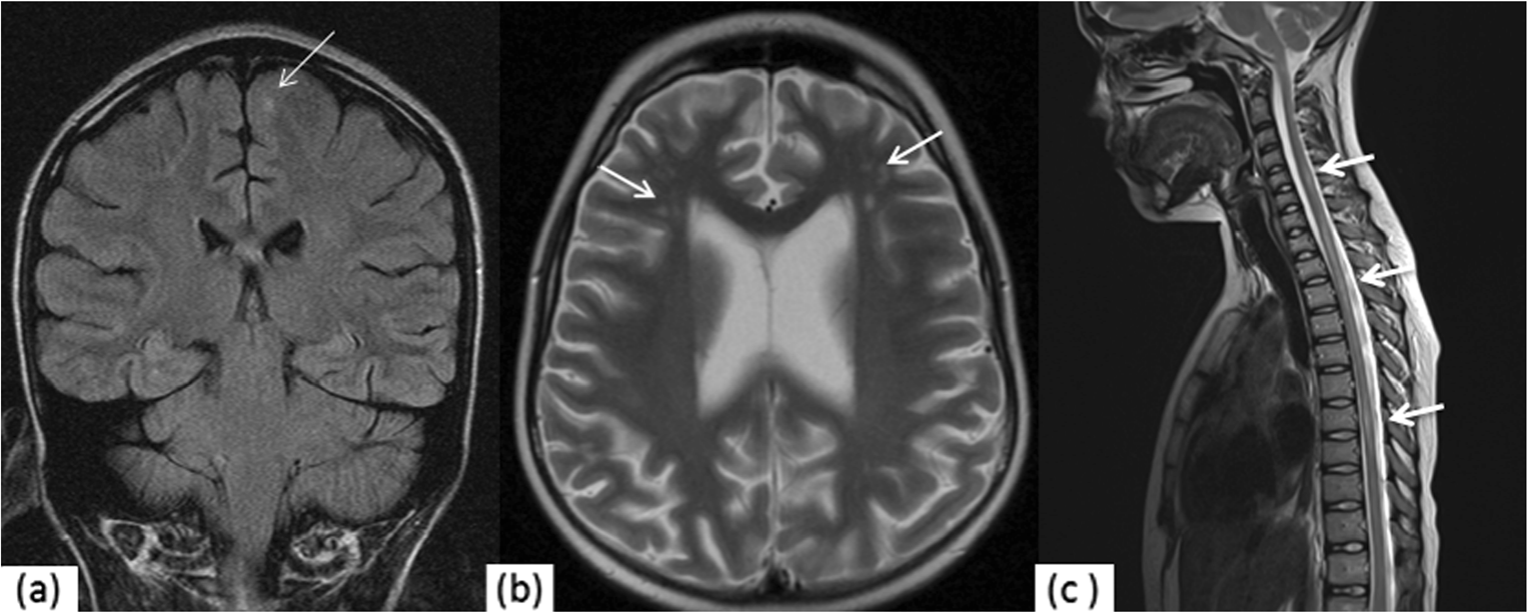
a Representative example of a grey matter signal hyperintensity (*arrow*) seen on the coronal FLAIR image (*long arrow*). b Clusters of T2 hyperintensities (*arrows*) in the frontal white matter seen on T2-weighted images. Note the background of sulcal and ventricular enlargement possibly resulting from cerebral atrophy. c Sagittal T2 hyperintensity of the cord (*arrows*) that resolved and remitted over a number of years. *FLAIR* fluid-attenuated inversion recovery
